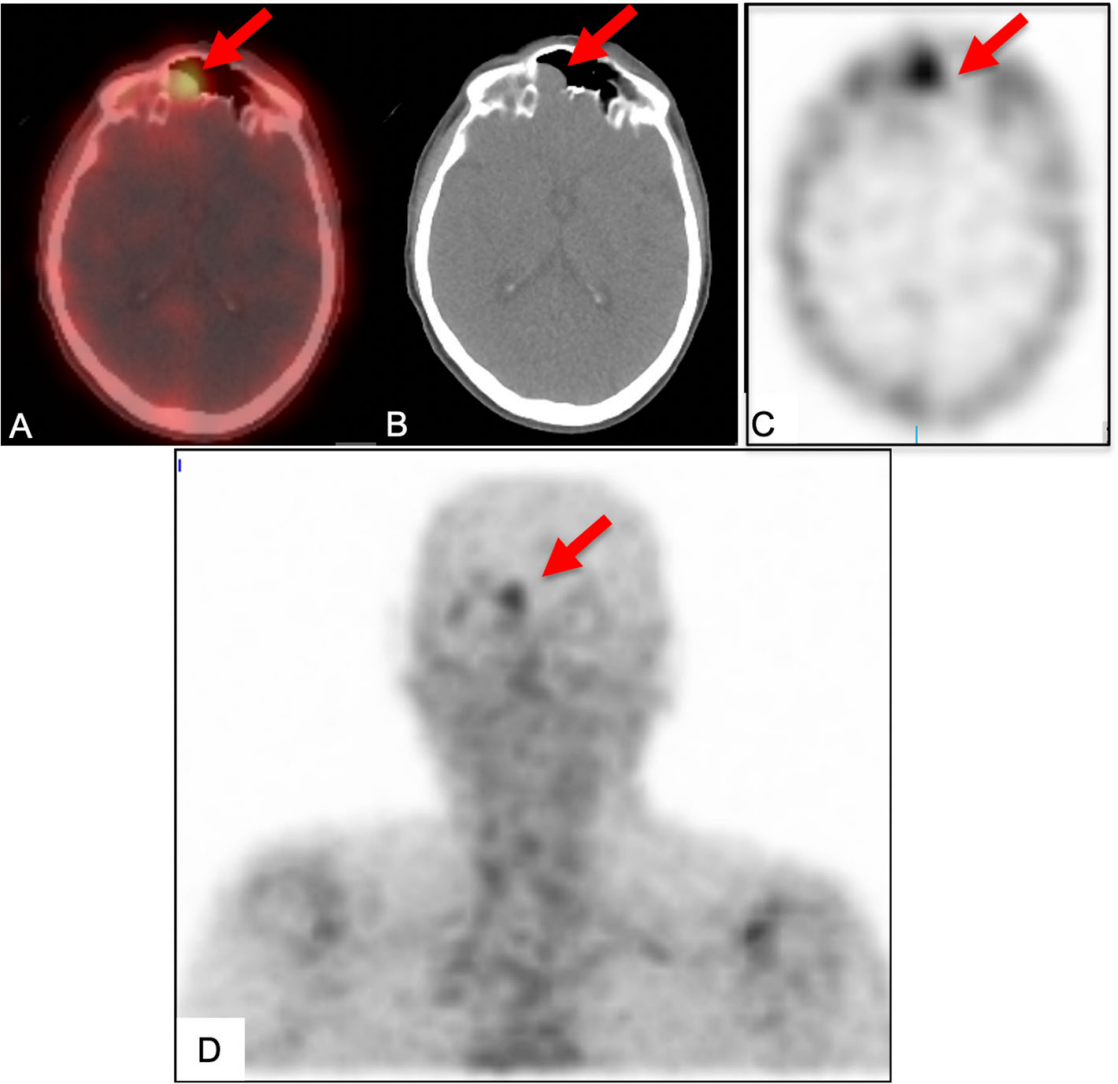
PET (a), CT (b), and octreotide scans (c, d) of patient in case 4, performed at the National Institute of Health, identified a 3 cm right frontal sinus tumor (red arrows) with bone erosion and abutment of the dura
